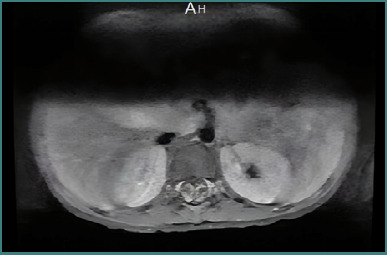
Axial T1-weighted gadolinium-enhanced MRI of the lumbar spine showing cauda equina nerve roots enhancement
